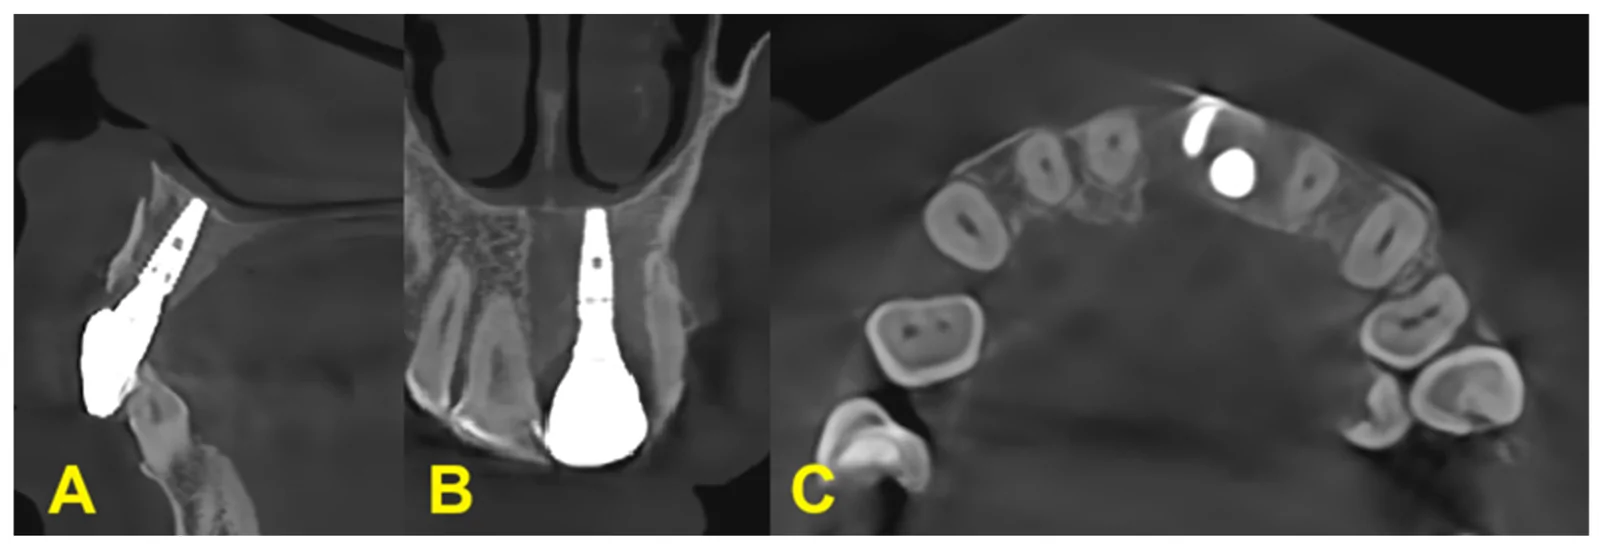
Cone-beam computed tomography of maxillary left incisor position after prosthetic crown delivery: (A) cross-sectional views; (B) coronal view; (C) axial view.
